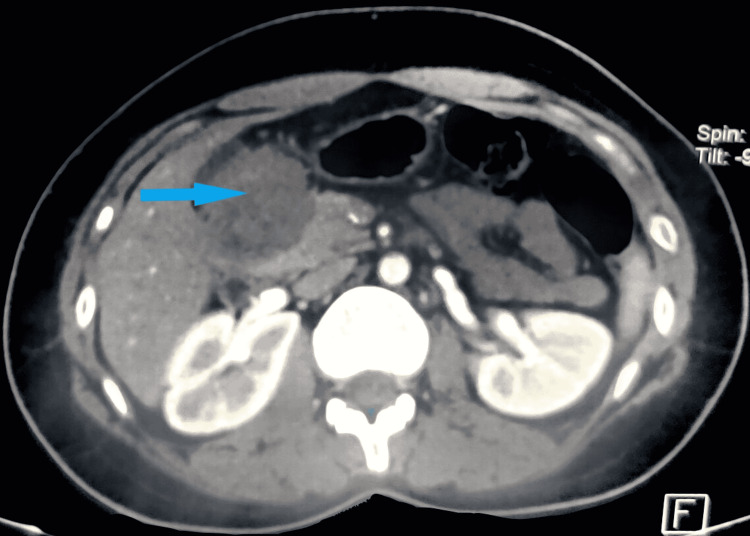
Contrast-enhanced computed tomography of the abdomen showing a well-defined mild heterogeneously enhancing soft tissue density measuring about 5.8 × 4 cm noted in the right subhepatic space around the pyloroduodenal junction (blue arrow)
